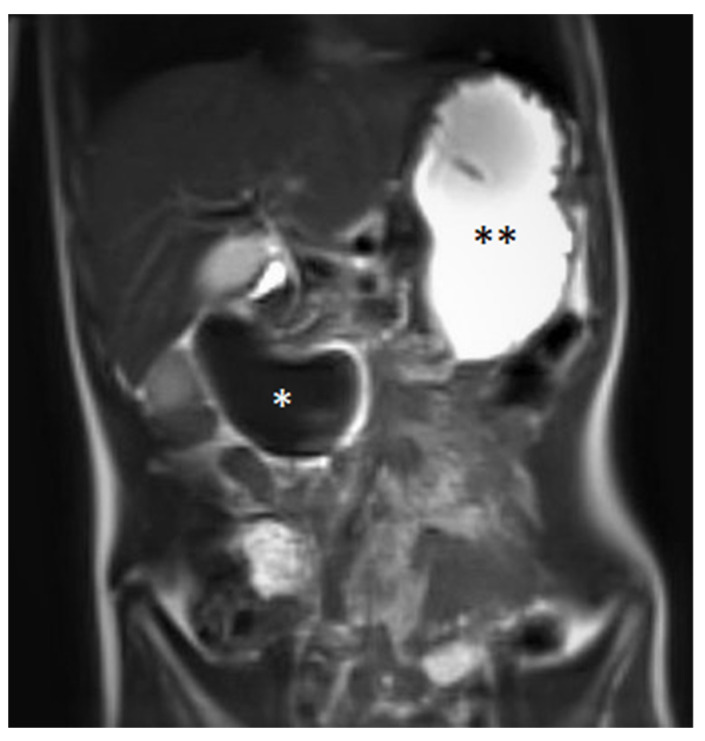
Coronal magnetic resonance imaging in the T2 HASTE sequence showing a large duodenal hematoma (*) and an ileus of the proximal small intestine with the filled stomach (). Image quality is reduced due to the patient being restless.
